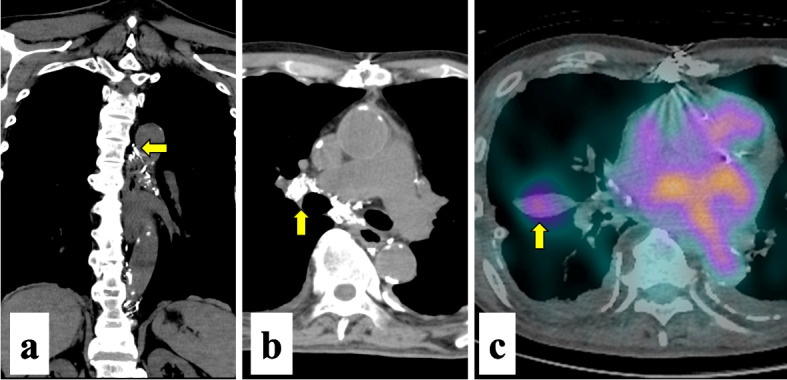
Mechanism of pericarditis-induced chylothorax. a Lymphangiography shows that the thoracic duct is interrupted at the Th5/6 level (arrow) by postoperative pericardial inflammation. b Delayed lymphangiography images showing that obstruction of the thoracic duct leads to development of lymphatic collaetarls via the tracheal bifurcation and right hilum (arrow). c Single-photon emission computed tomography showing abnormal tracer accumulation in the right hilum between the middle and lower lobes (arrow), suggesting a lymphatic leakage from ruptured lymphatic collaterals
